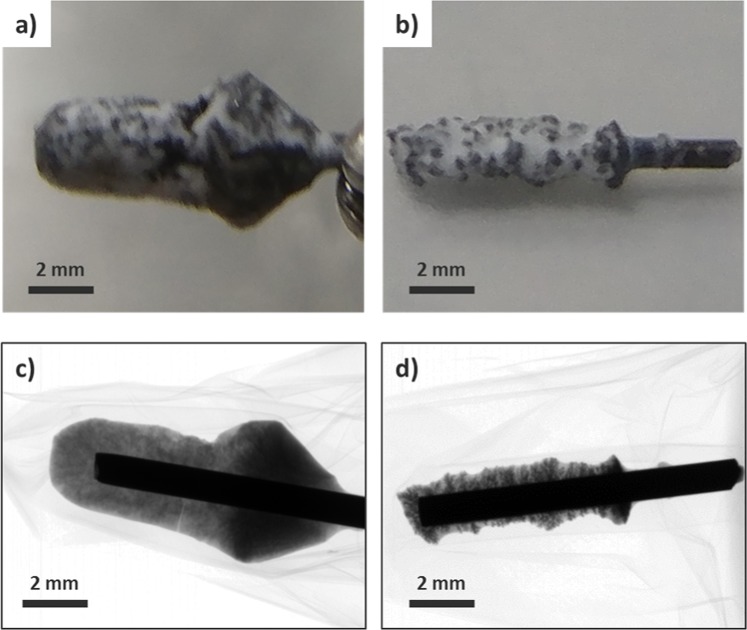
(a) Photograph of electrodeposit obtained by CA at −2.05 V for 600 seconds. (b) Photograph of electrodeposit obtained by CP at −2 mA for 16,500 seconds. (c,d) Their radiographic images (rotational view: Supplementary Videos S4 and S5), both 33 coulombs (current (I) × time (s)) of charge flow.
